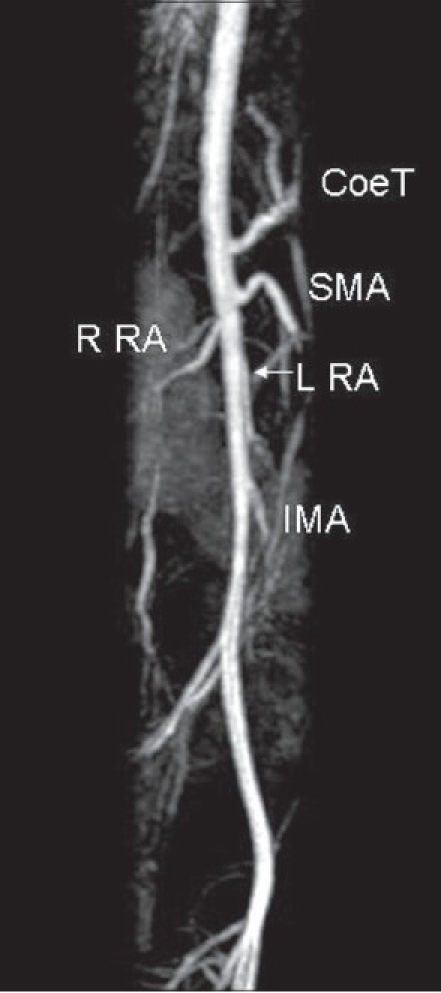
Major Branches of Abdominal Aorta (MRI). R RA – Right Renal Artery, L RA - Left Renal Artery
